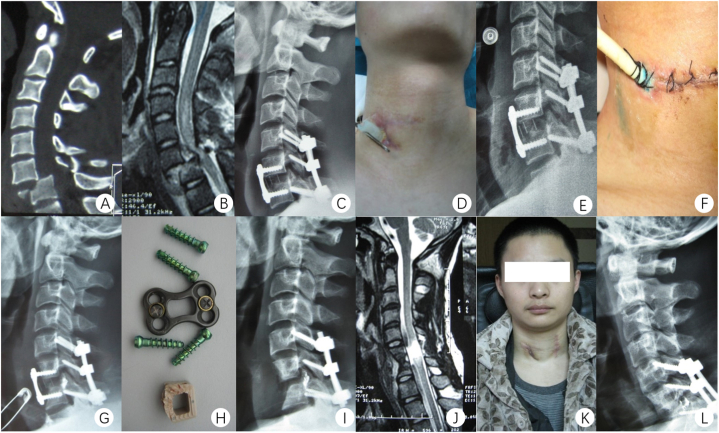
A 22-year-old male presented with a cervical fracture and dislocation (C6/7) leading to quadriplegia, classified as ASIA D. Preoperative CT (A). Preoperative MRI (B). Postoperative DR (C). Photograph of the neck incision 2 months post-surgery (D). DR at 2 months post-surgery (E). Image of incision after oral methylene blue test (F). DR at 5 months after debridement (G). A picture of the removed internal fixation (H). DR after removal of internal fixation (I). MRI 1 week after the esophageal repair (J). General picture of the patient (L). DR 5 months after the esophageal repair (K). (For interpretation of the references to colour in this figure legend, the reader is referred to the Web version of this article.)
